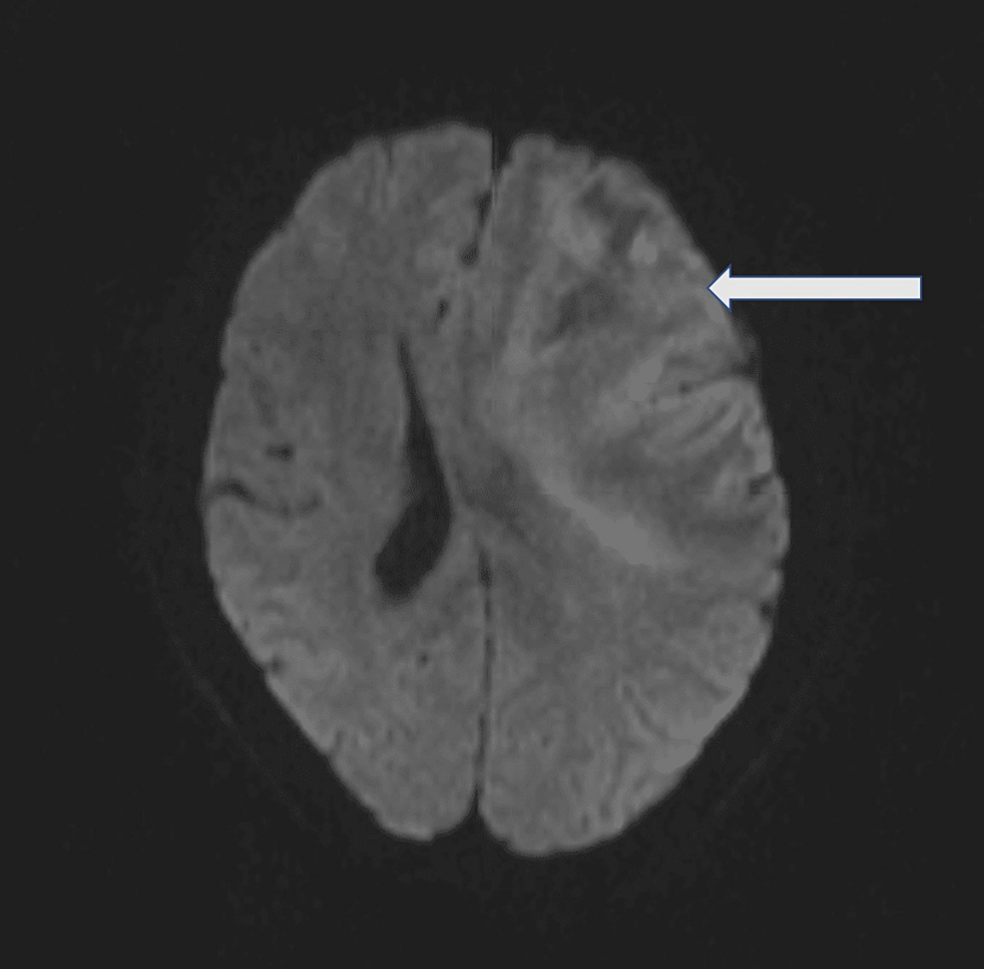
Diffusion-weighted imaging. The magnetic resonance imaging shows diffusion restriction in the left middle cerebral artery territory.
